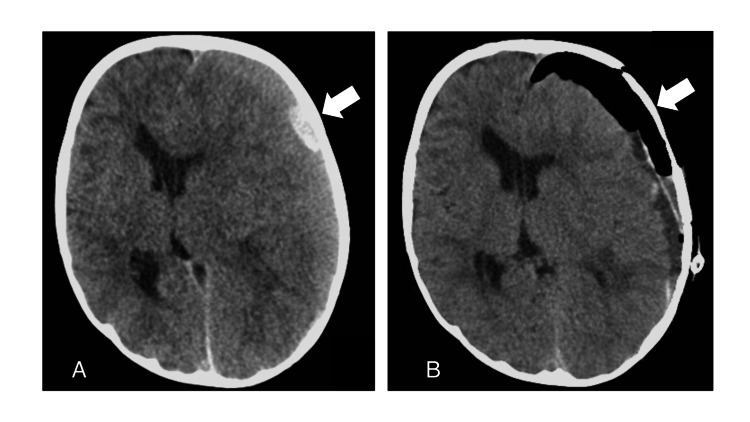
Preoperative and postoperative CT of the head (A) Preoperative head CT  showing acute and chronic subdural hematoma on the left side with midline shift; (B) Head CT just after hematoma removal surgery
